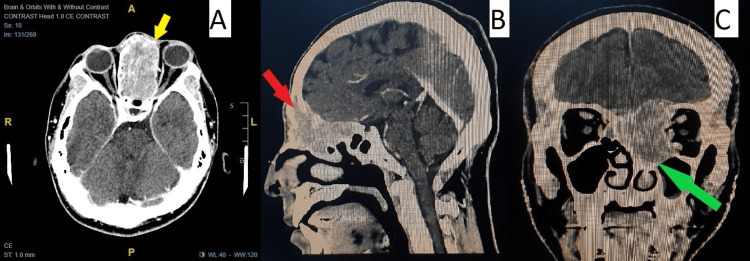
(A) Axial CECT (yellow arrow) shows a huge and highly aggressive mass from the ethmoid sinus extending to the left extraconal fat and stretching the left medial rectus muscle, abutting the intracanalicular part of the optic nerve. (B) Sagittal CECT (red arrow) shows destruction of the frontal bone, posterior wall of the frontal sinus, and cribriform plate with extension to the frontal sinus. Intracranial extension to the anterior cranial fossa was also noted. (C) Coronal CECT (green arrow) shows mass extending to the sphenoid sinus, maxillary sinus, and nasal cavity till the left middle meatus with disruption of the nasal bone. CECT, contrasted enhanced computed tomography
